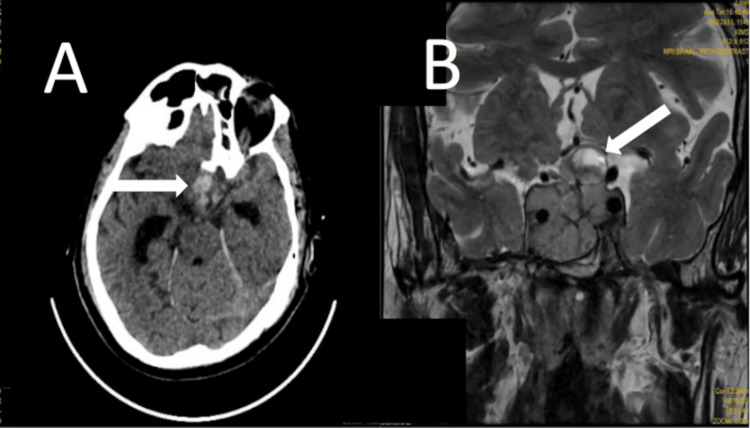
Case 5: (A) Computed tomography scan (axial section) done in the emergency department showing pituitary haemorrhage (arrow) after starting cabergoline therapy. (B) MRI T2-weighted image (coronal section) showing giant prolactinoma (arrow) with pituitary apoplexy.
